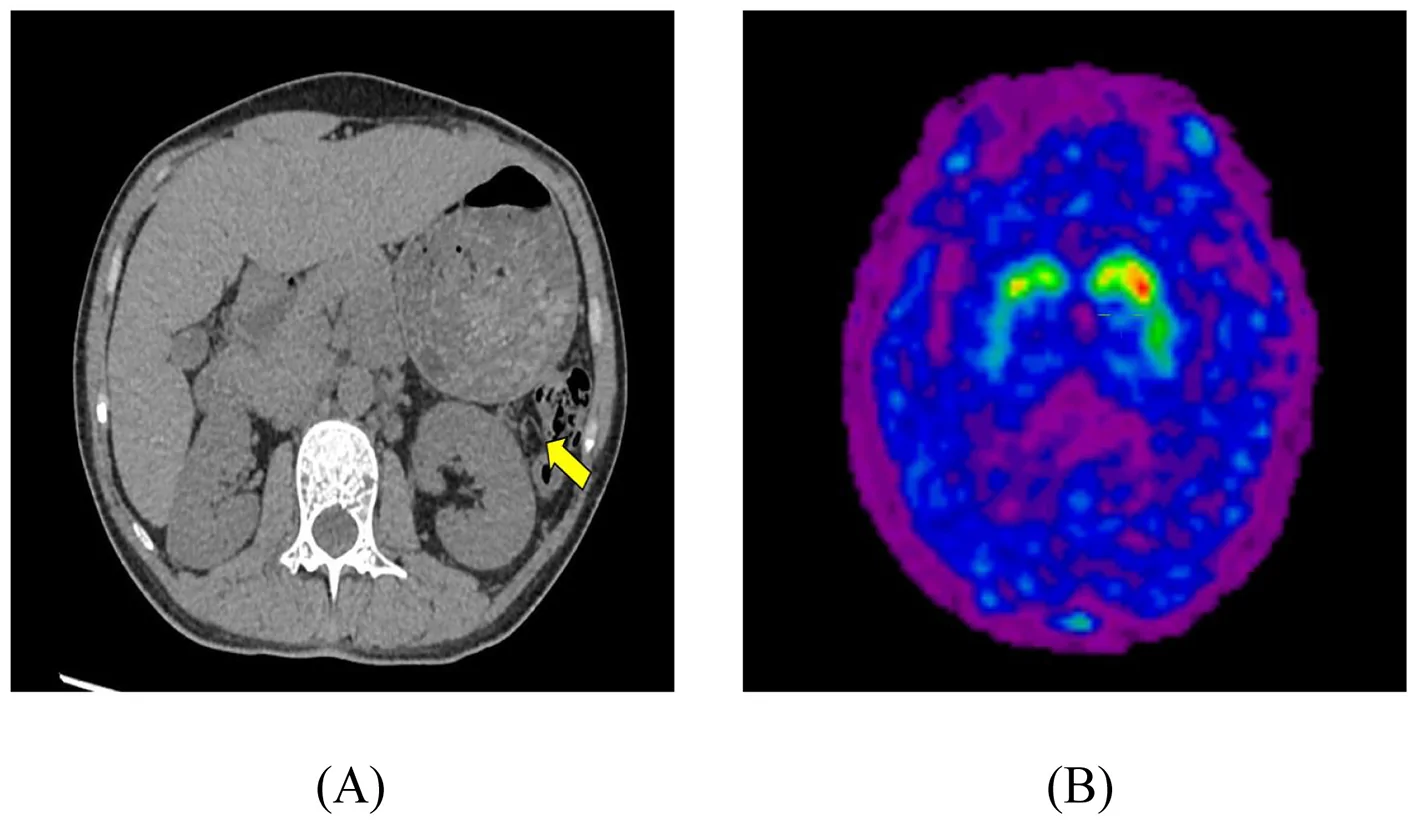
Multimodal imaging findings. (A) Abdominal CT showing absence of the spleen (arrow). (B) Dopamine transporter PET/CT demonstrating reduced radiotracer uptake in the bilateral putamen, more pronounced on the right.
